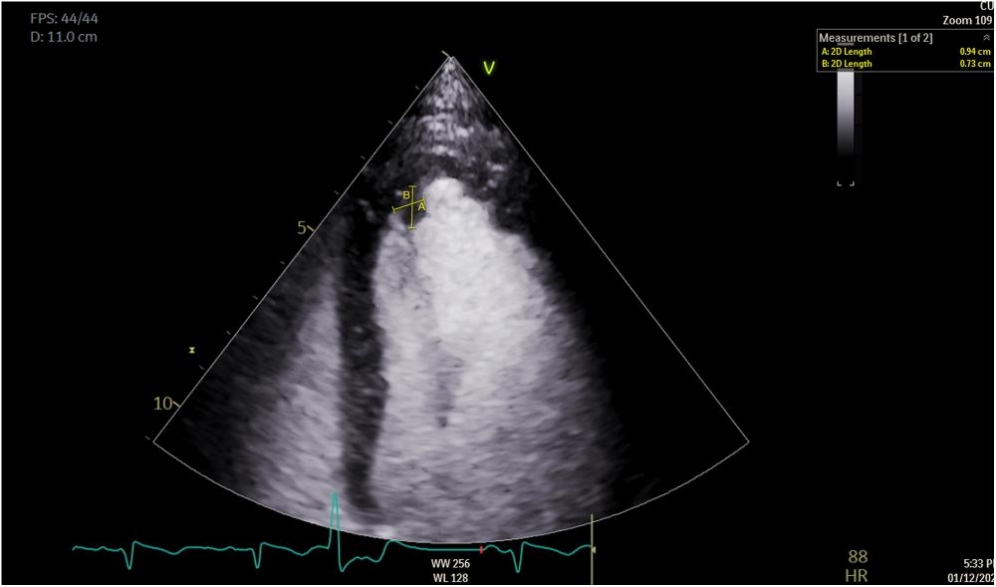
Focused contrast-echocardiography revealing small residual apical thrombus.
